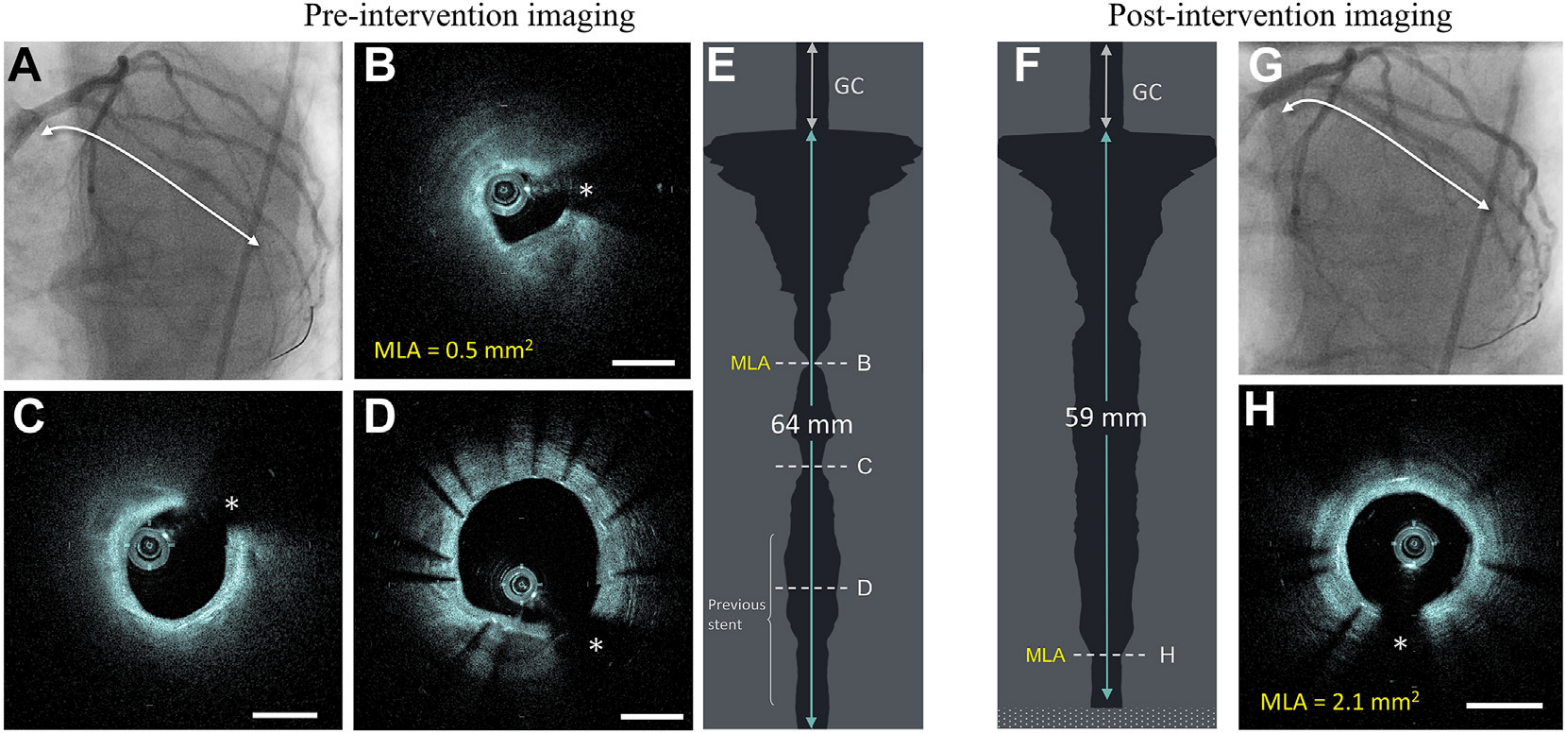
(A) The image showsx-ray angiography of a previously stented left circumflex artery preintervention. (B) The high-frequency optical coherence tomography (HF-OCT) cross-shows an MLA equal to 0.5 mm^2^ located proximally to the stented region. (C) The image shows a second, more distal, luminal narrowing. (D) HF-OCT imaging of the previous stent, showing a thin layer of neointimal tissue. (E) Despite the tight lumen area, HF-OCT images free of blood were obtained, including the vessel segment distal to the MLA. (F) The image shows the lumen profile image postintervention. (G) The x-ray image shows postintervention angiographic results. (H) The HF-OCT image shows the MLA site postintervention. The asterisk indicates the guide wire shadowing artifact. Scale bars are equal to 1 mm. GC, guide catheter; MLA, minimum lumen area.
